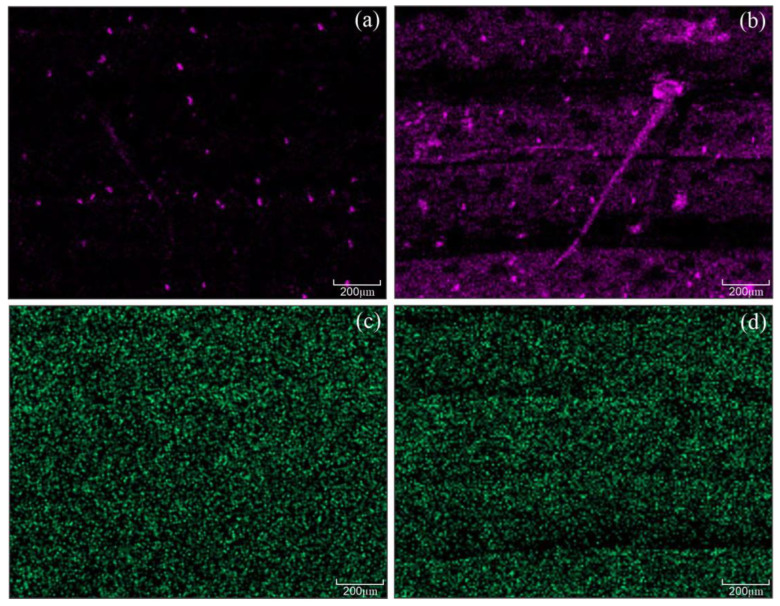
X-ray maps for the presence of silicon (Si) (a,b) and potassium (K) (c,d) in the leaves of maize plants non-supplied (−Si) (a and c) or supplied (+Si) (b and d) with Si at 156 h after inoculation with *Bipolaris maydis* (isolate UFV-DPF-*Bm*12). In the X-ray maps, purple (a,b) and green (c,d) fluorescent dots correspond to the accumulation of Si or K, respectively. Black indicates the absence of Si or K. Scale bars = 200 μm.
